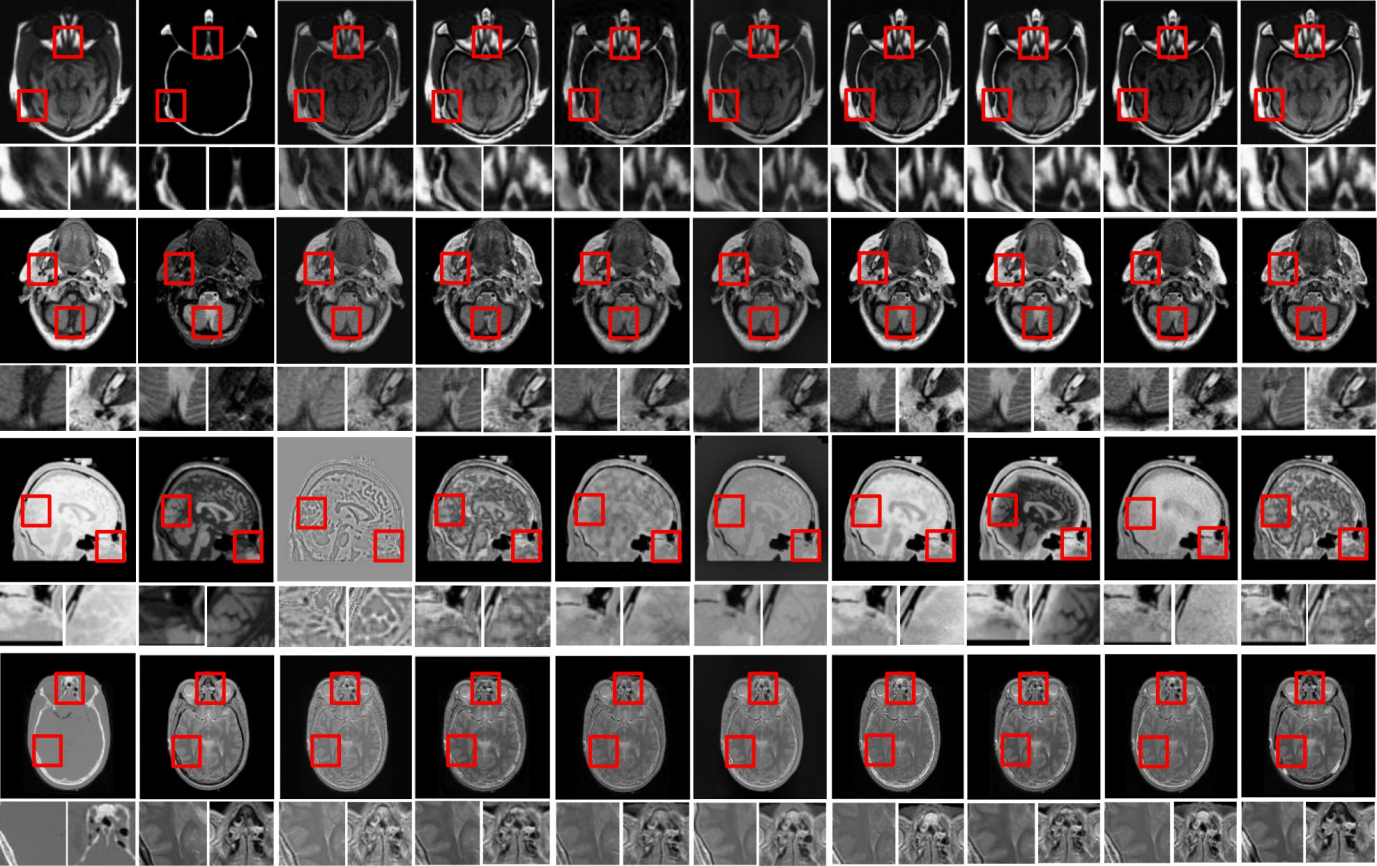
Qualitative comparisons of the proposed algorithm with 7 state-of-the-art algorithms in 4 representative CT-MRI image pairs. From left to right: CT image, MRI image, fusion results of SGF-SR [30], MODE-DNN [33], CNN-NSCT [59], DGF [37],SR-SCNN [35], FDGNet [41], DFENet [42] and the proposed method. For best analysis, two local regions are zoomed as close-ups in each image.
